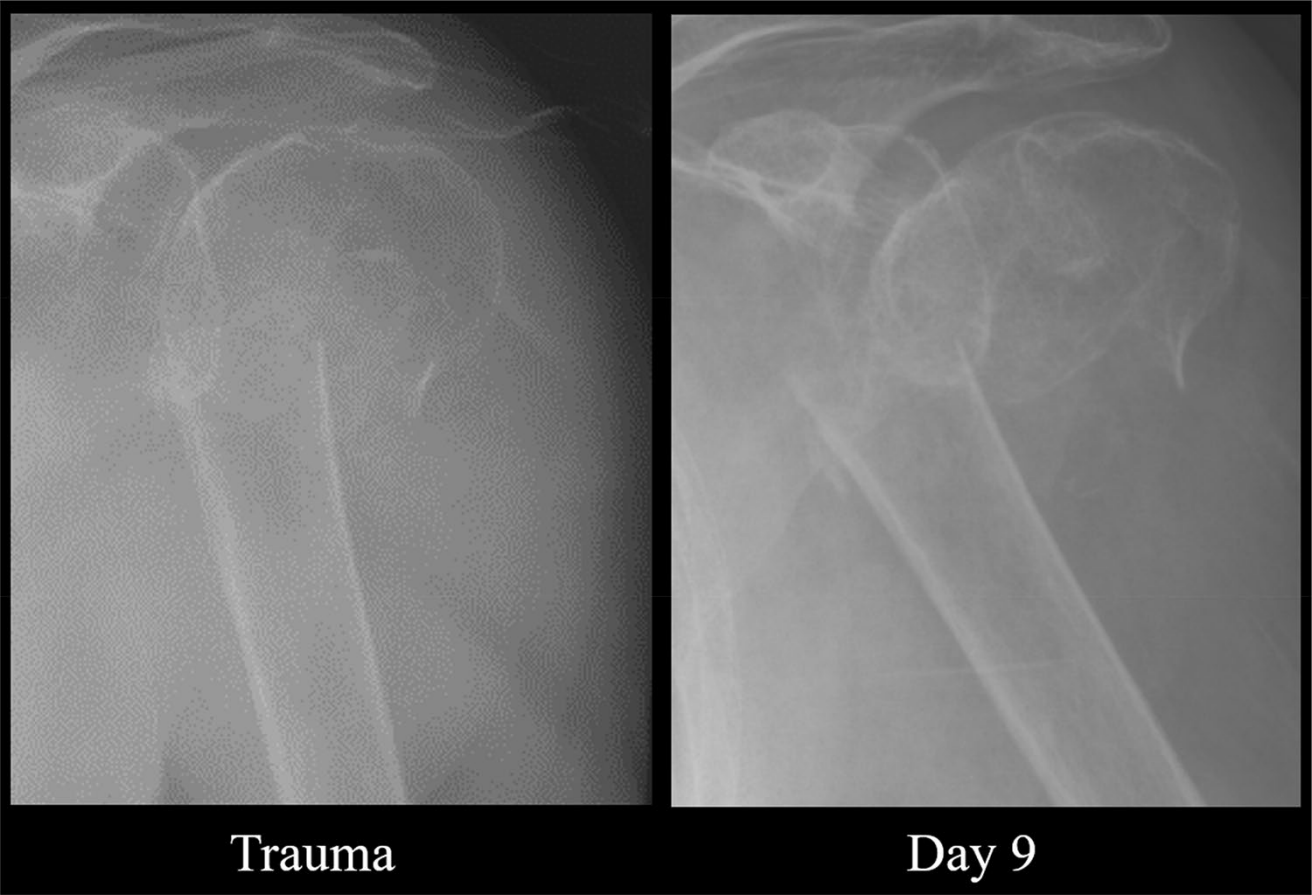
Radiographic follow-up of a type B fracture. Trauma: NSA = 146.4°, MMG = 24.9 mm, MLG = 18.6 mm. Day 9: NSA = 152.2°, MMG = 34.4 mm, MLG = 33.5 mm
